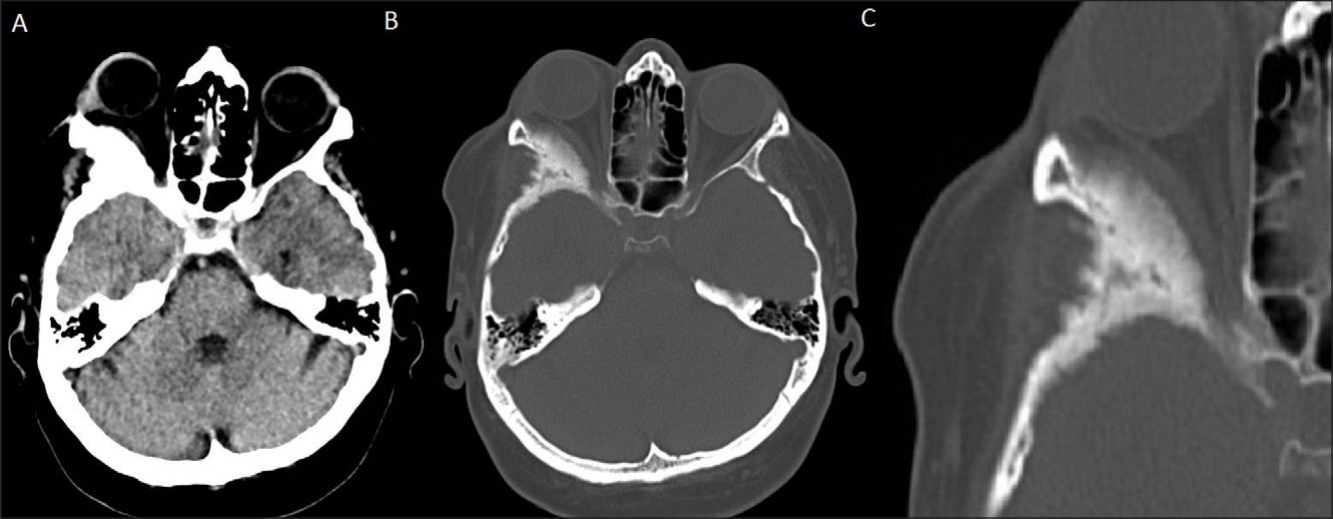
Non-contrast enhanced axial CT of the brain with soft tissue (A) and bony window reconstructions (B) shows an expansile sclerotic lesion in the right sphenoid wing. Involvement of the right lateral orbital wall leads to narrowing of the orbital space and secondary proptosis. A zoomed-in view in bony window (C) shows the irregularly marginated sclerotic expansion of the right lateral orbital wall in more detail.
